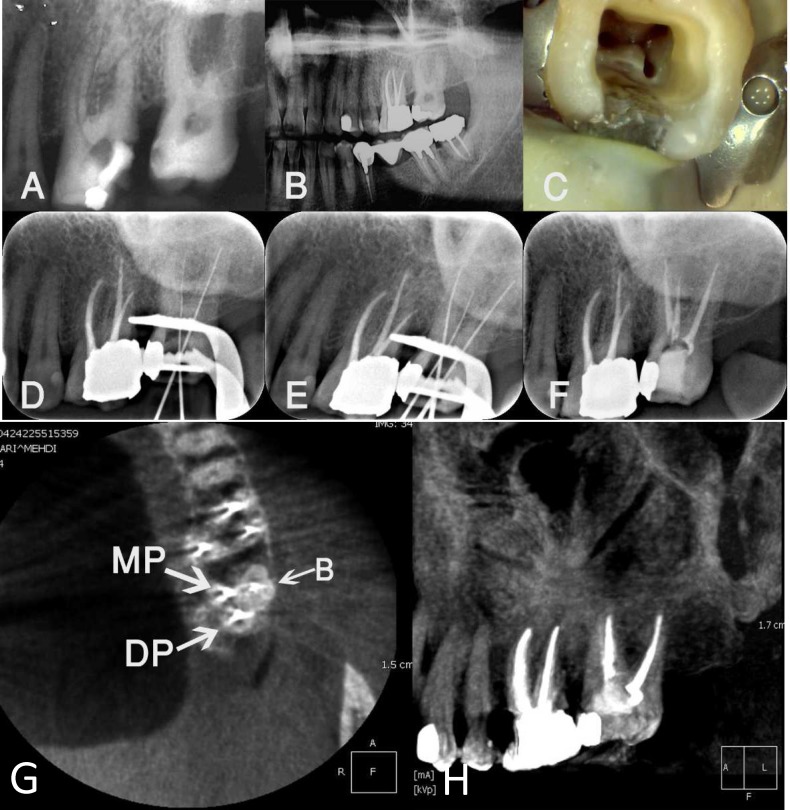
Pre-operative periapical radiography of the maxillary left second molar before restoration with amalgam;* B)* Panoramic view of the tooth after amalgam placement and following episodes of pain;* C)* Detecting buccal and distobuccal root canals after file placement and taking radiography;* D) *Root canal orifices of the tooth; one orifice is on the buccal and there are orifices to two palatal root canals;* E)* Radiograph taken after detecting all root canals;* F)* Periapical radiography after completion of the root canal filling;* G)* Axial CBCT view;* H)* Sagittal CBCT view*
